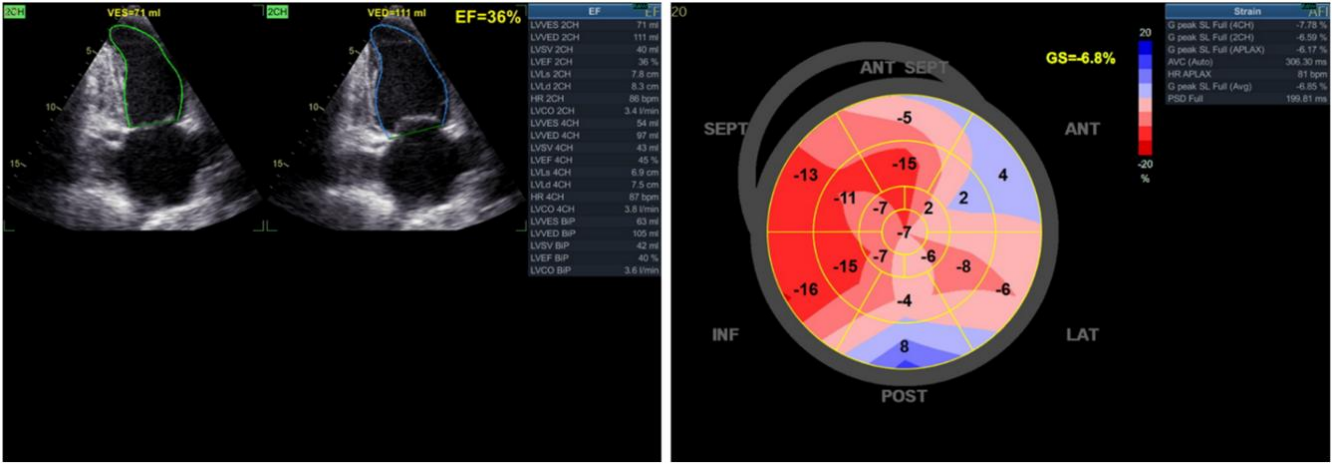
Echocardiography revealed an improvement in left ventricular contractile function (increasing from 14% to 36%). However, the global longitudinal strain (GLS) index on speckle tracking echocardiography remained low at −6.8%, suggesting injury in the anterior wall.
